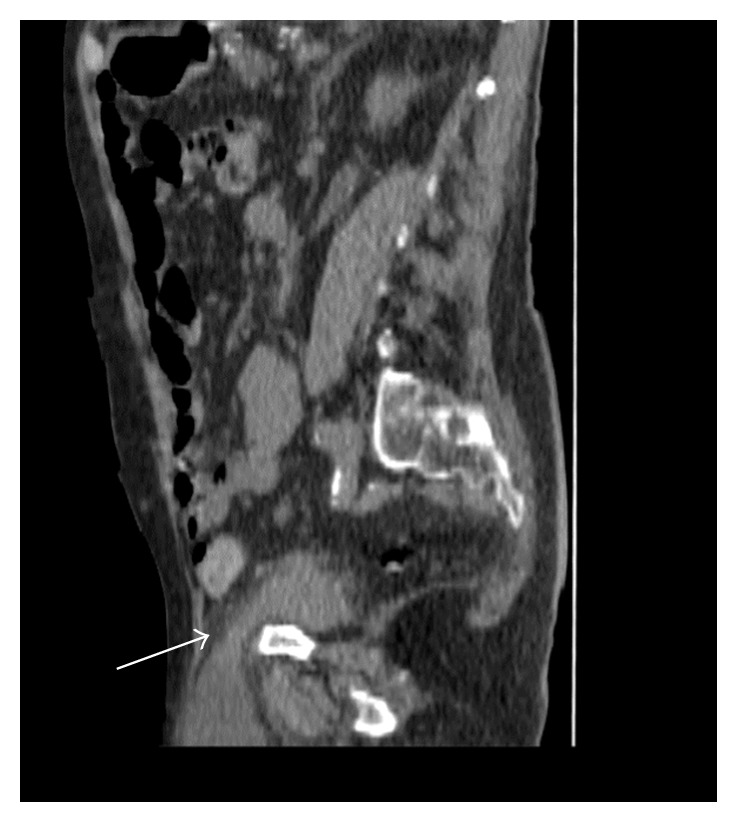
Sagittal CT section showing bladder herniation.
